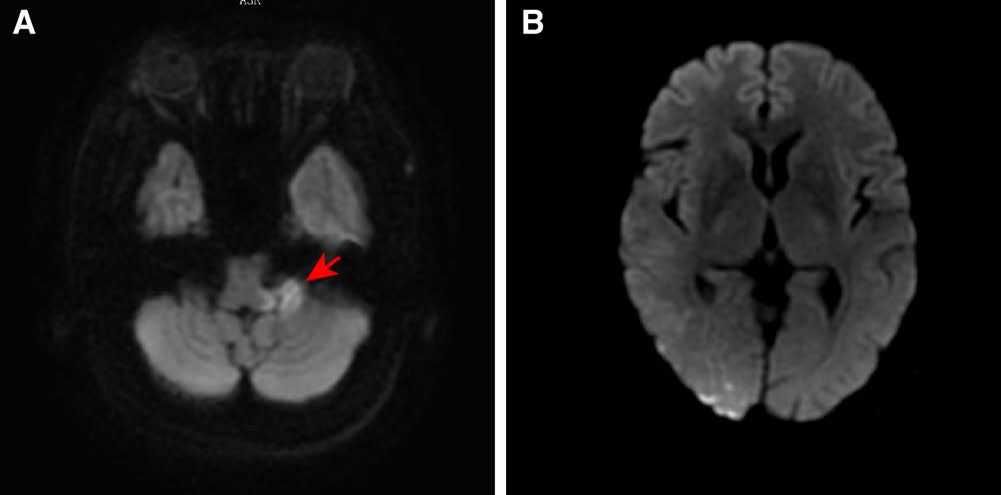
Diffusion-weighted imaging (DWI) in the brain. (A) A high-intensity signal involving the left middle cerebellar peduncle; (B) several spotted areas in the right occipital lobe.
